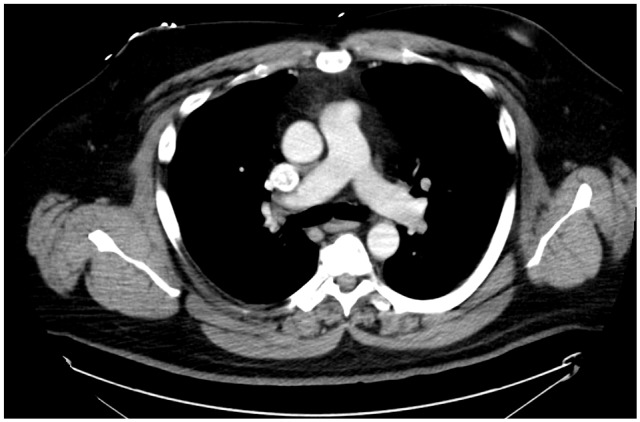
Computed tomography scan of chest with contrast. No pulmonary embolism.
